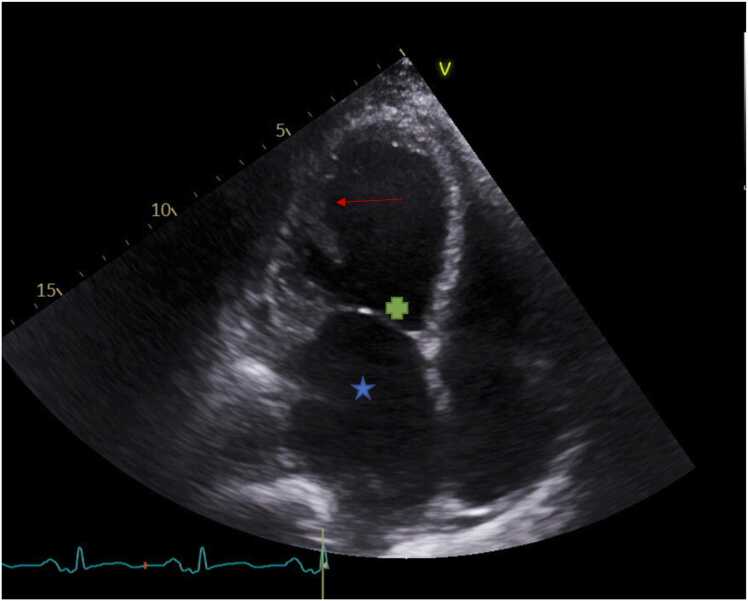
Apical 4-chamber view during transthoracic echocardiography. *Red arrow*: inside LV cavity pointing to crypts within LV-free wall; *: left atrium; +: mitral valve (closed). LV, left ventricle.
